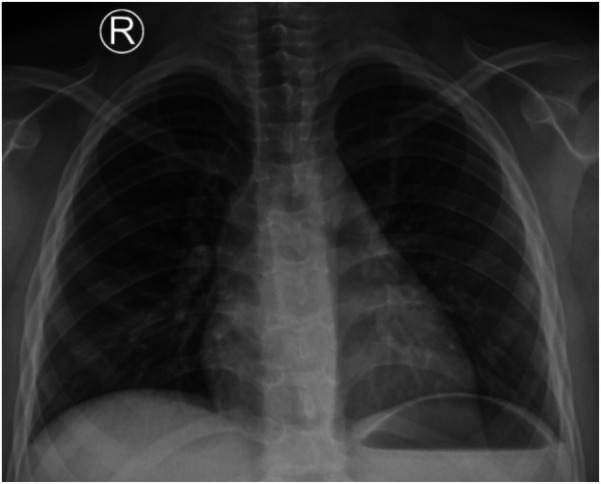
Chest x-ray of the child on the day of admission.
